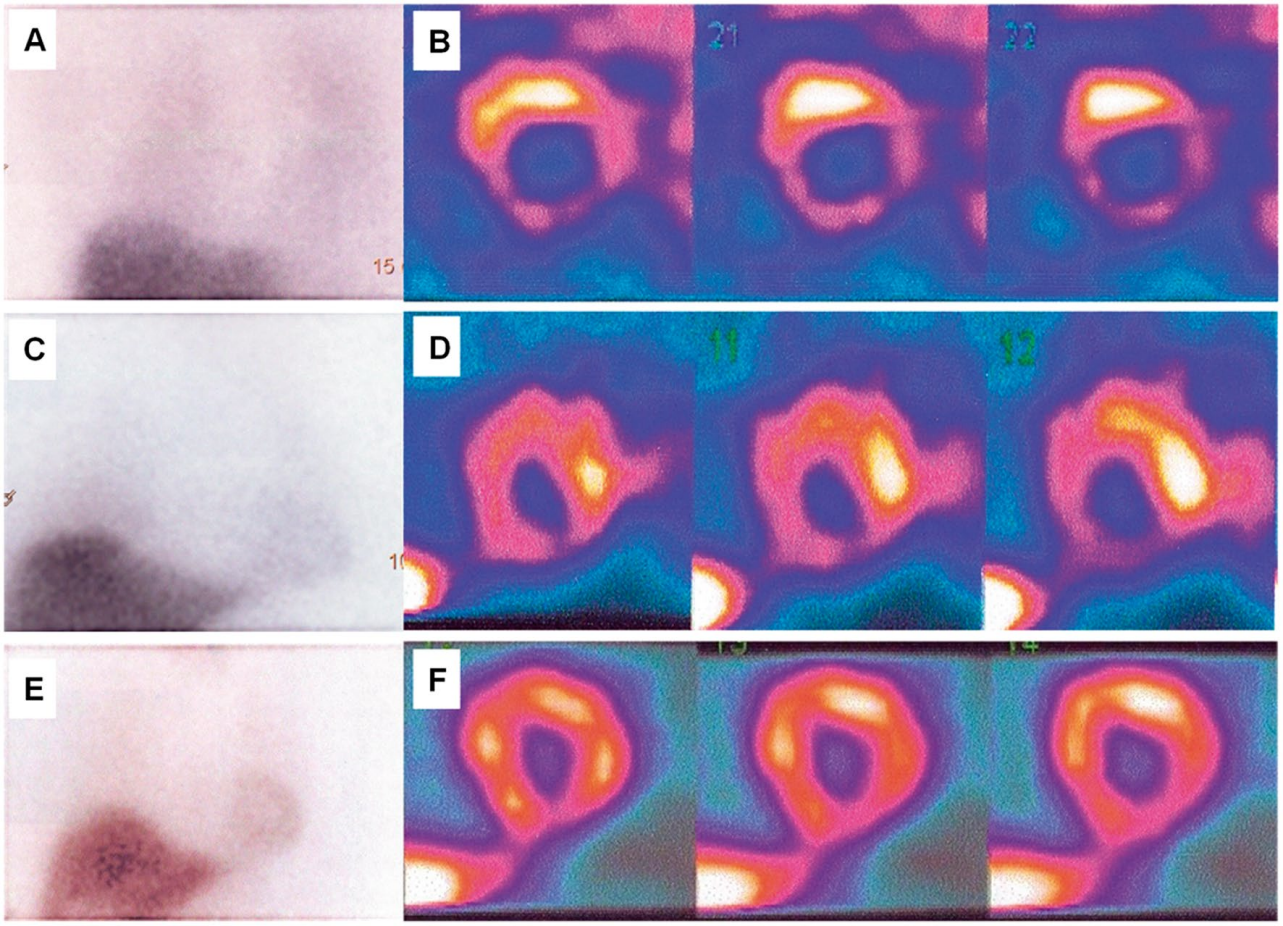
Planar ^123^I-MIBG late images (a, c, e) and selected short-axis slices (b, d, f) from SPECT late acquisition. (a, b) Reduced late heart to mediastinum ratio (1.3) and a clear apical defect on SPECT images. (c, d) Mild reduction of the heart to mediastinum ratio (1.5) and a slight apical defect on SPECT images. (e, f) Normal heart to mediastinum ratio (1.8) and normal distribution of radiotracer on SPECT images. ^123^I-MIBG, ^123^I-metaiodobenzyl-guanidine; SPECT, single-photon emission computed tomography. (Reprinted with permission from Citro et al. [26])
